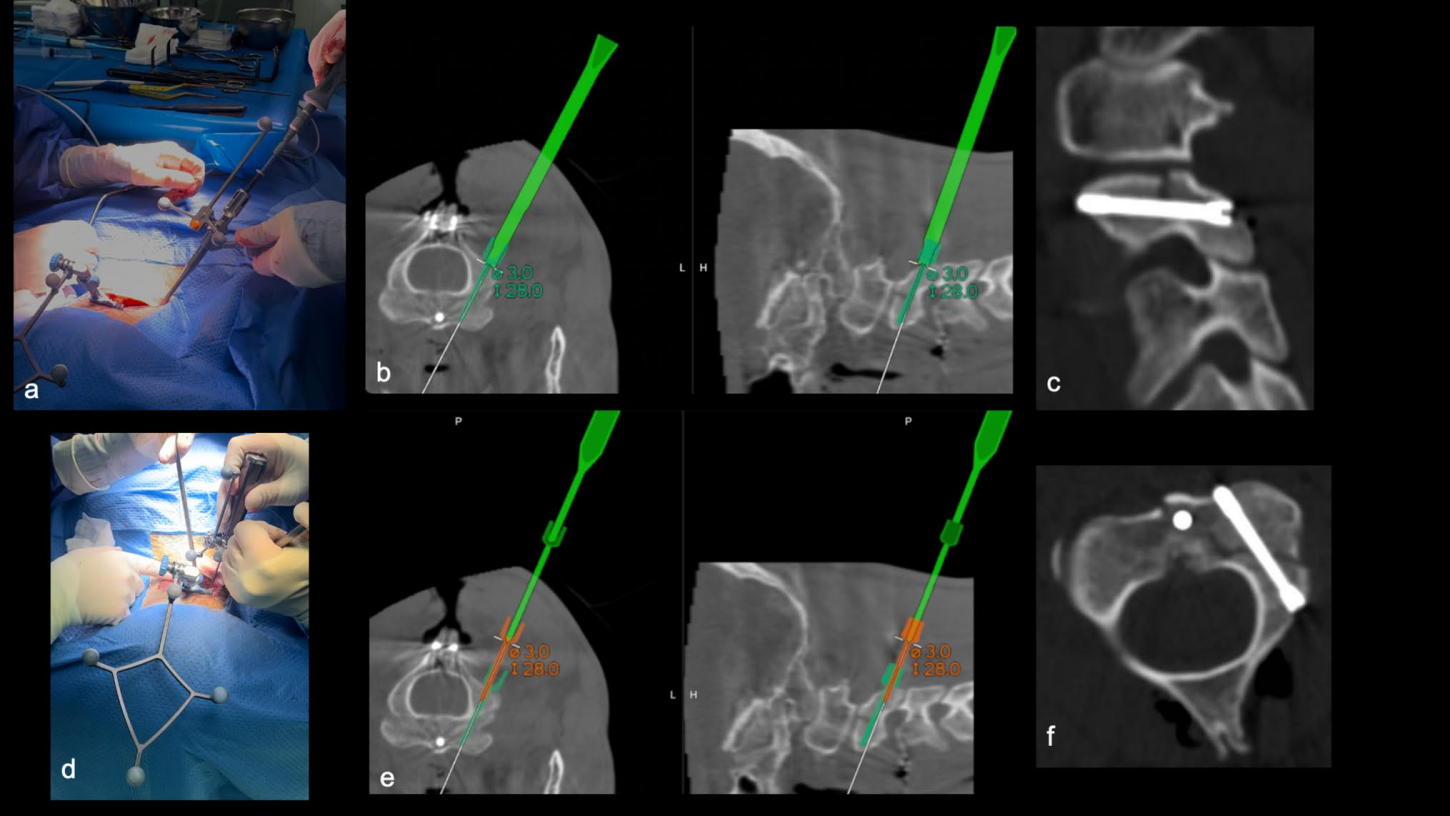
a Intraosseous drilling is performed through a neuronavigated working channel, guided in real-time by the neuronavigation system; b the trajectory follows the pre-planned path. d A navigated screw holder is used to place the screw, again guided by the pre-planned imaging (e). Intraoperative cervical spine CT images confirm the correct positioning of the screw in the left pedicle of C2, shown in sagittal (c) and axial (f) views
